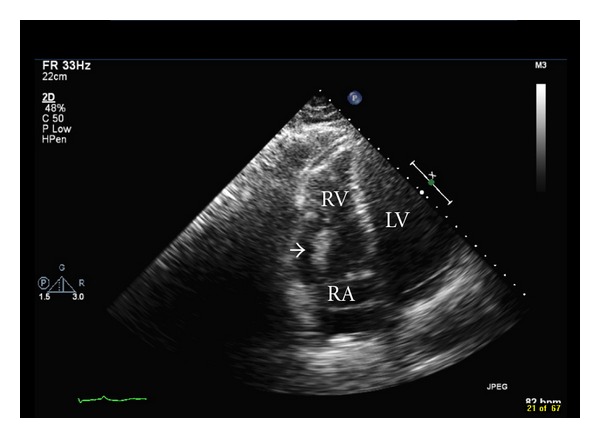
Transthoracic echocardiography showing a large right atrial thrombus (white arrow) extending into the right ventricle.
